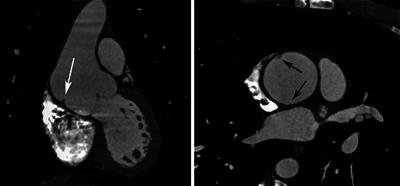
Aortic CTA showing ascending aorta dilatation with an intraluminal parietal band.
